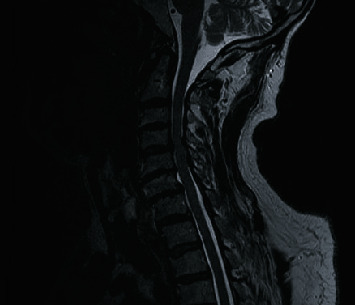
Sagittal T2-weighted MRI of the cervical spine, demonstrating spinal cord compression at the level of C4-C5.
